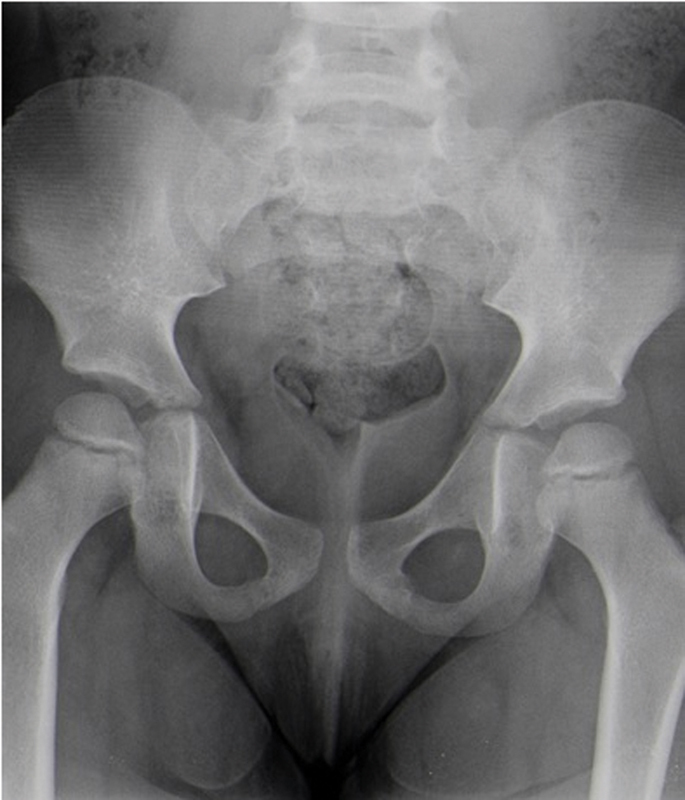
Radiography of the pelvis (anteroposterior view), revealing complete recovery.
